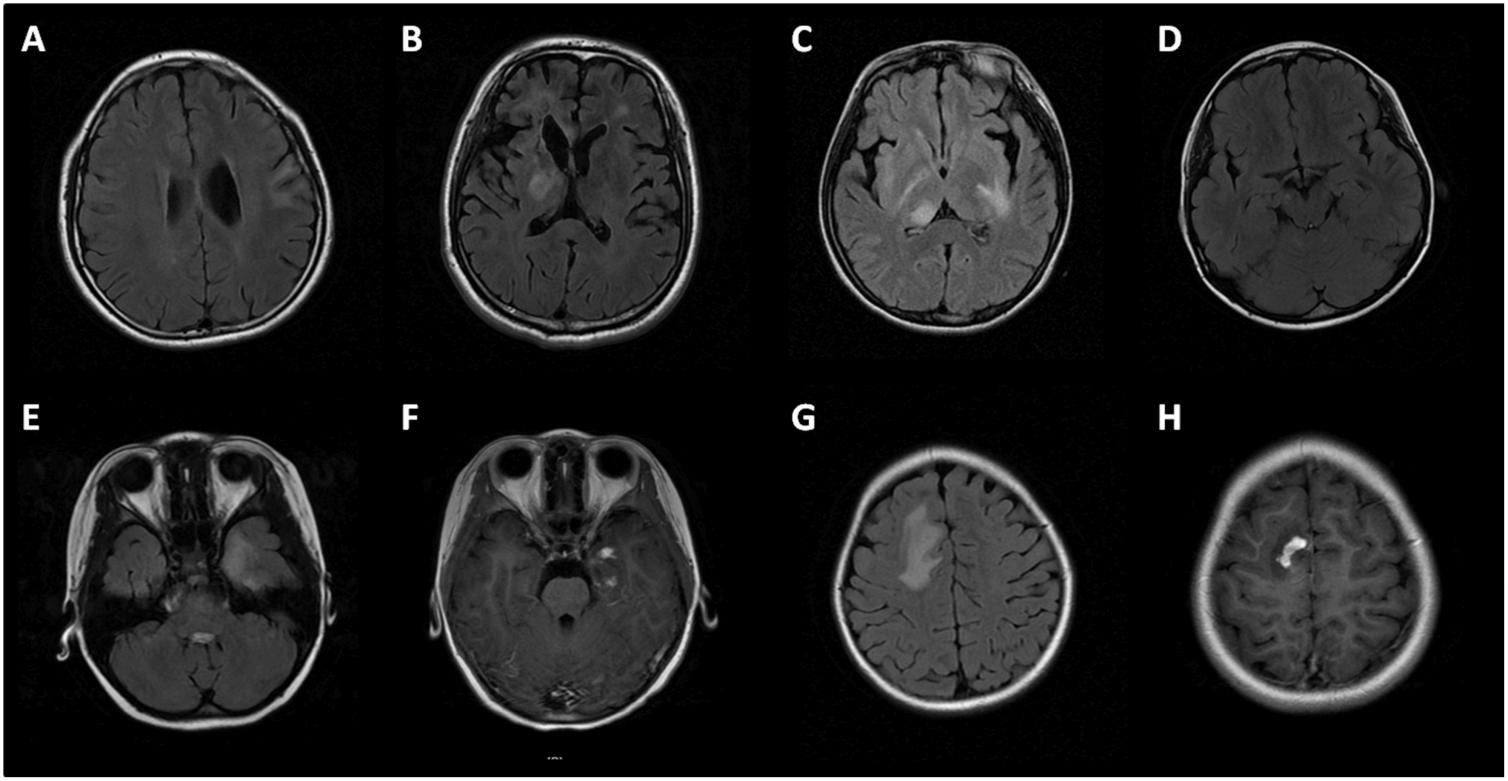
Brain MRI of MOG-IgG positive patients with seizures and/or encephalopathy. (A) Cortical and subcortical lesions; (B) Deep white matter lesions and periventricular lesions; (C) Thalamus lesions; (D) Cerebral peduncle lesions; (E,G) Large white matter lesions of a patient in temporal lobe and parietal lobe, with prominent gadolinium enhancement (F,H).
